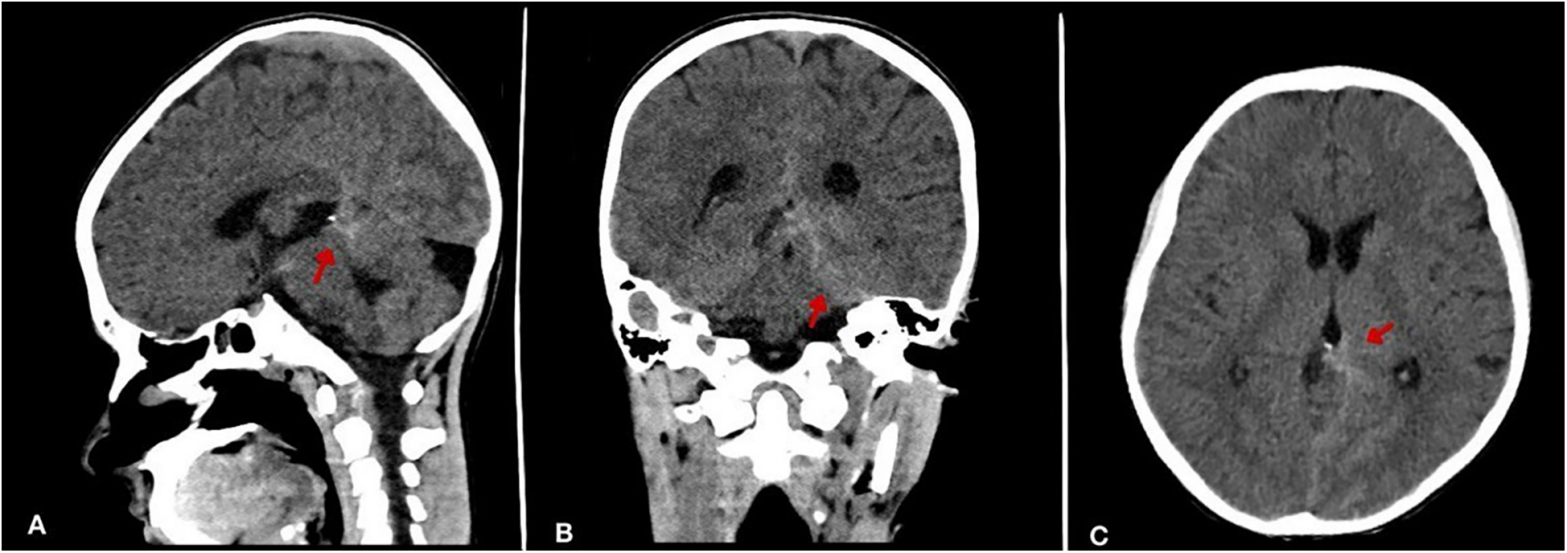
Non-contrast brain CT demonstrating subarachnoid hemorrhage (SAH). (A) Sagittal view shows hyperdensity (arrow) within the interhemispheric fissure and basal cisterns. (B) Coronal view reveals prominent hemorrhage (arrow) in perimesencephalic cisterns. (C) Axial section confirms diffuse hyperdensity (arrow) in basal cisterns.
